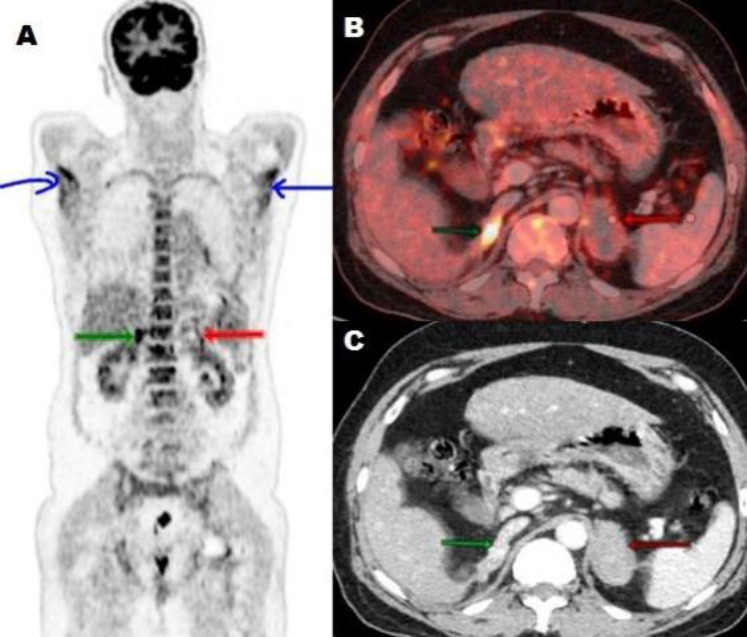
(A) Whole body ^18^F-FDG PET maximum intensity projection image shows increased uptake in the bilateral suprarenal regions (arrows) and in the shoulder/scapular regions. (B) Fused axial PET/CT and (C) corresponding contrast enhanced CT images show an FDG avid left adrenal lesion with central necrosis and an FDG avid enhancing right adrenal lesion
